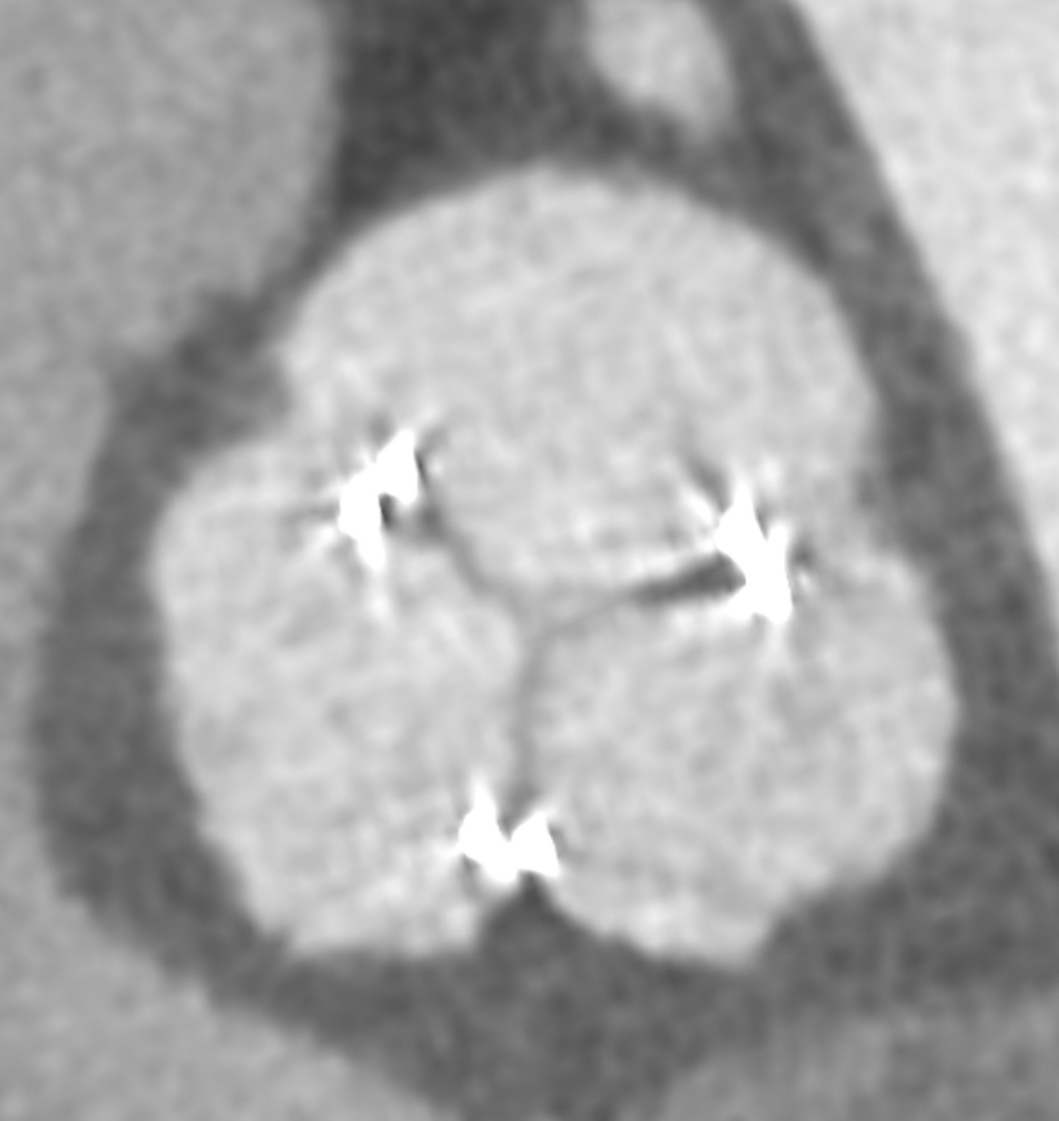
Postoperative CT shows no thrombus in bioprosthetic aortic valve.
